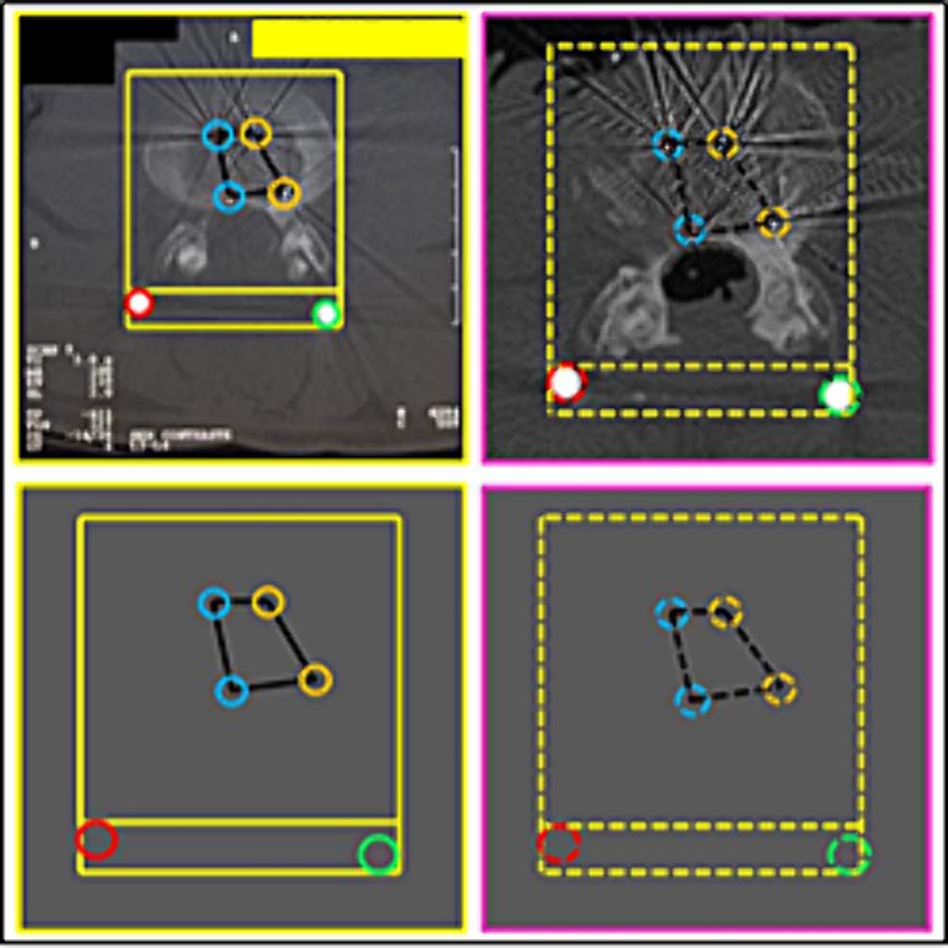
L3 vertebral body. Upper panels, comparison between the axial slices of the antemortem (left) and postmortem (right) CT scans. Lower panels, respective concordant templates.
